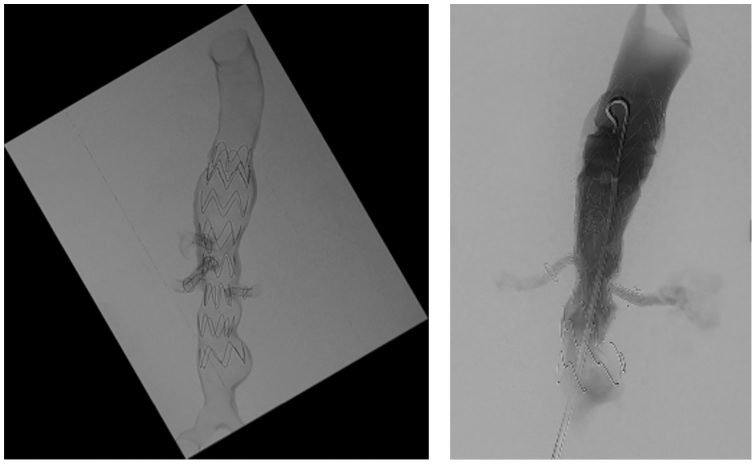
Completion single shot radiograph confirming stent deployment in all four visceral vessels (*left*) and completion angiogram demonstrating vessel patency (*right*).
